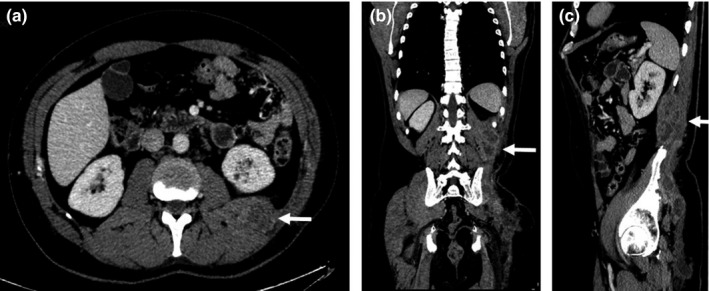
Computed tomography images showing an inhomogenous mass in the left flank of the patient extending through the retroperitoneal fat toward the peri‐renal space (white arrows) in axial (a), coronal (b), and sagittal (c) views.
